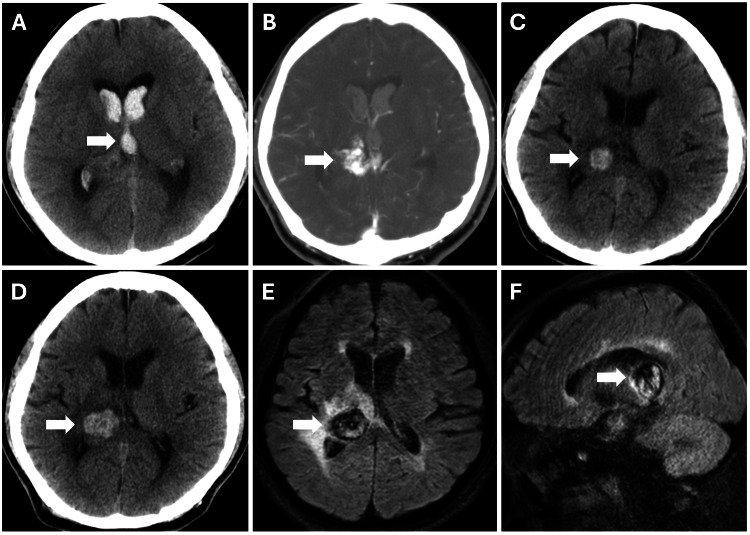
Initial imaging findings (Case 2) Computed tomography (CT) demonstrating an intraventricular hemorrhage (IVH) 14 years previously (A, arrow). CT angiography shows a right thalamic arteriovenous malformation as the cause of IVH (B arrow). CT showing a right thalamic hemorrhage with a maximum diameter of 1.4 cm (C arrow), which increased to 2.4 cm two months later (D arrow). Fluid-attenuated inversion recovery magnetic resonance imaging showing a reticulated pattern of mixed hyperintensity, hypointensity, and mild edematous changes around the hemorrhage (E and F, arrows).
